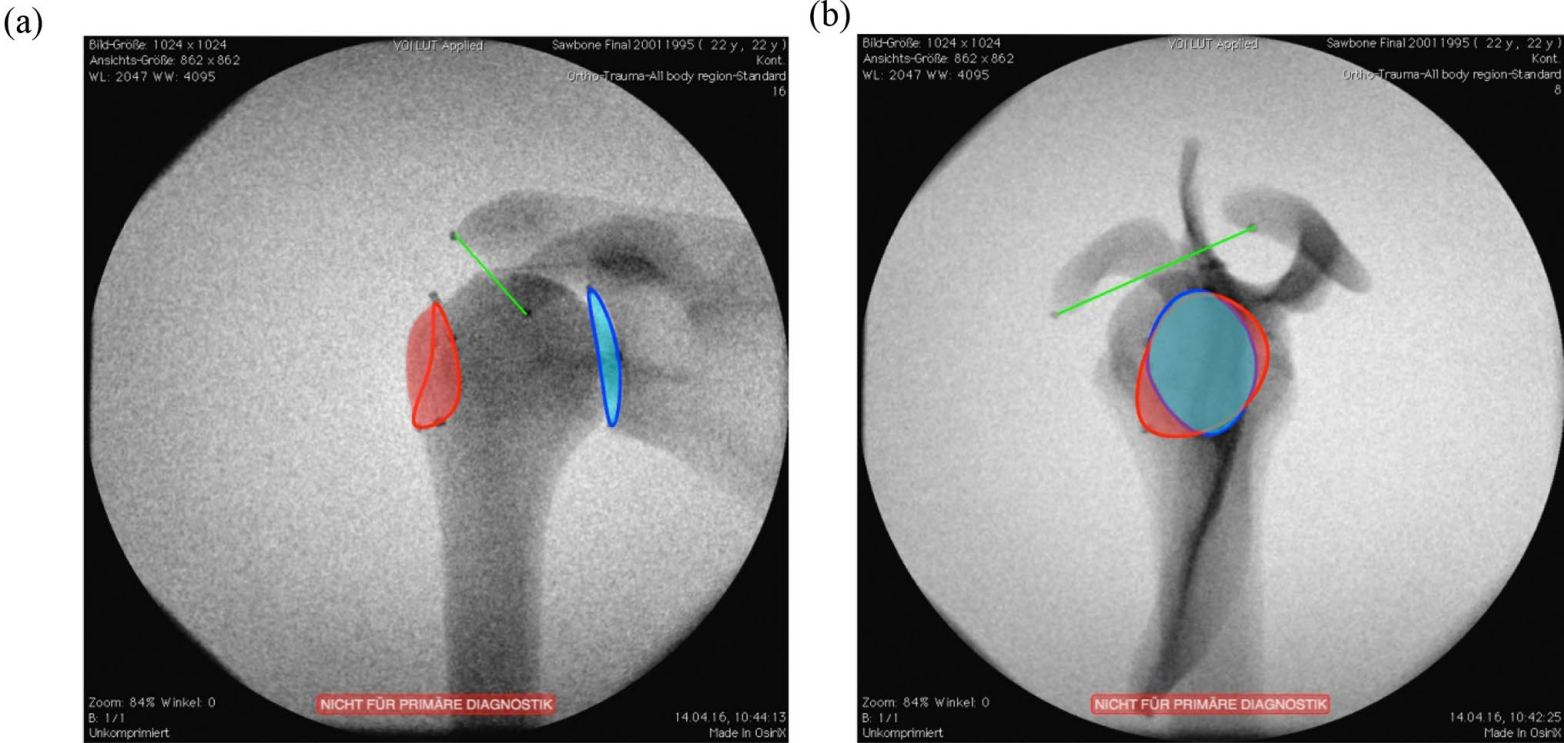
Two of the 36 radiographs of the saw bone shoulder: a anteroposterior and b scapular Y-views showing the greater tuberosity (red), glenoid (blue) and distance between the acromion and coracoid process (green)
